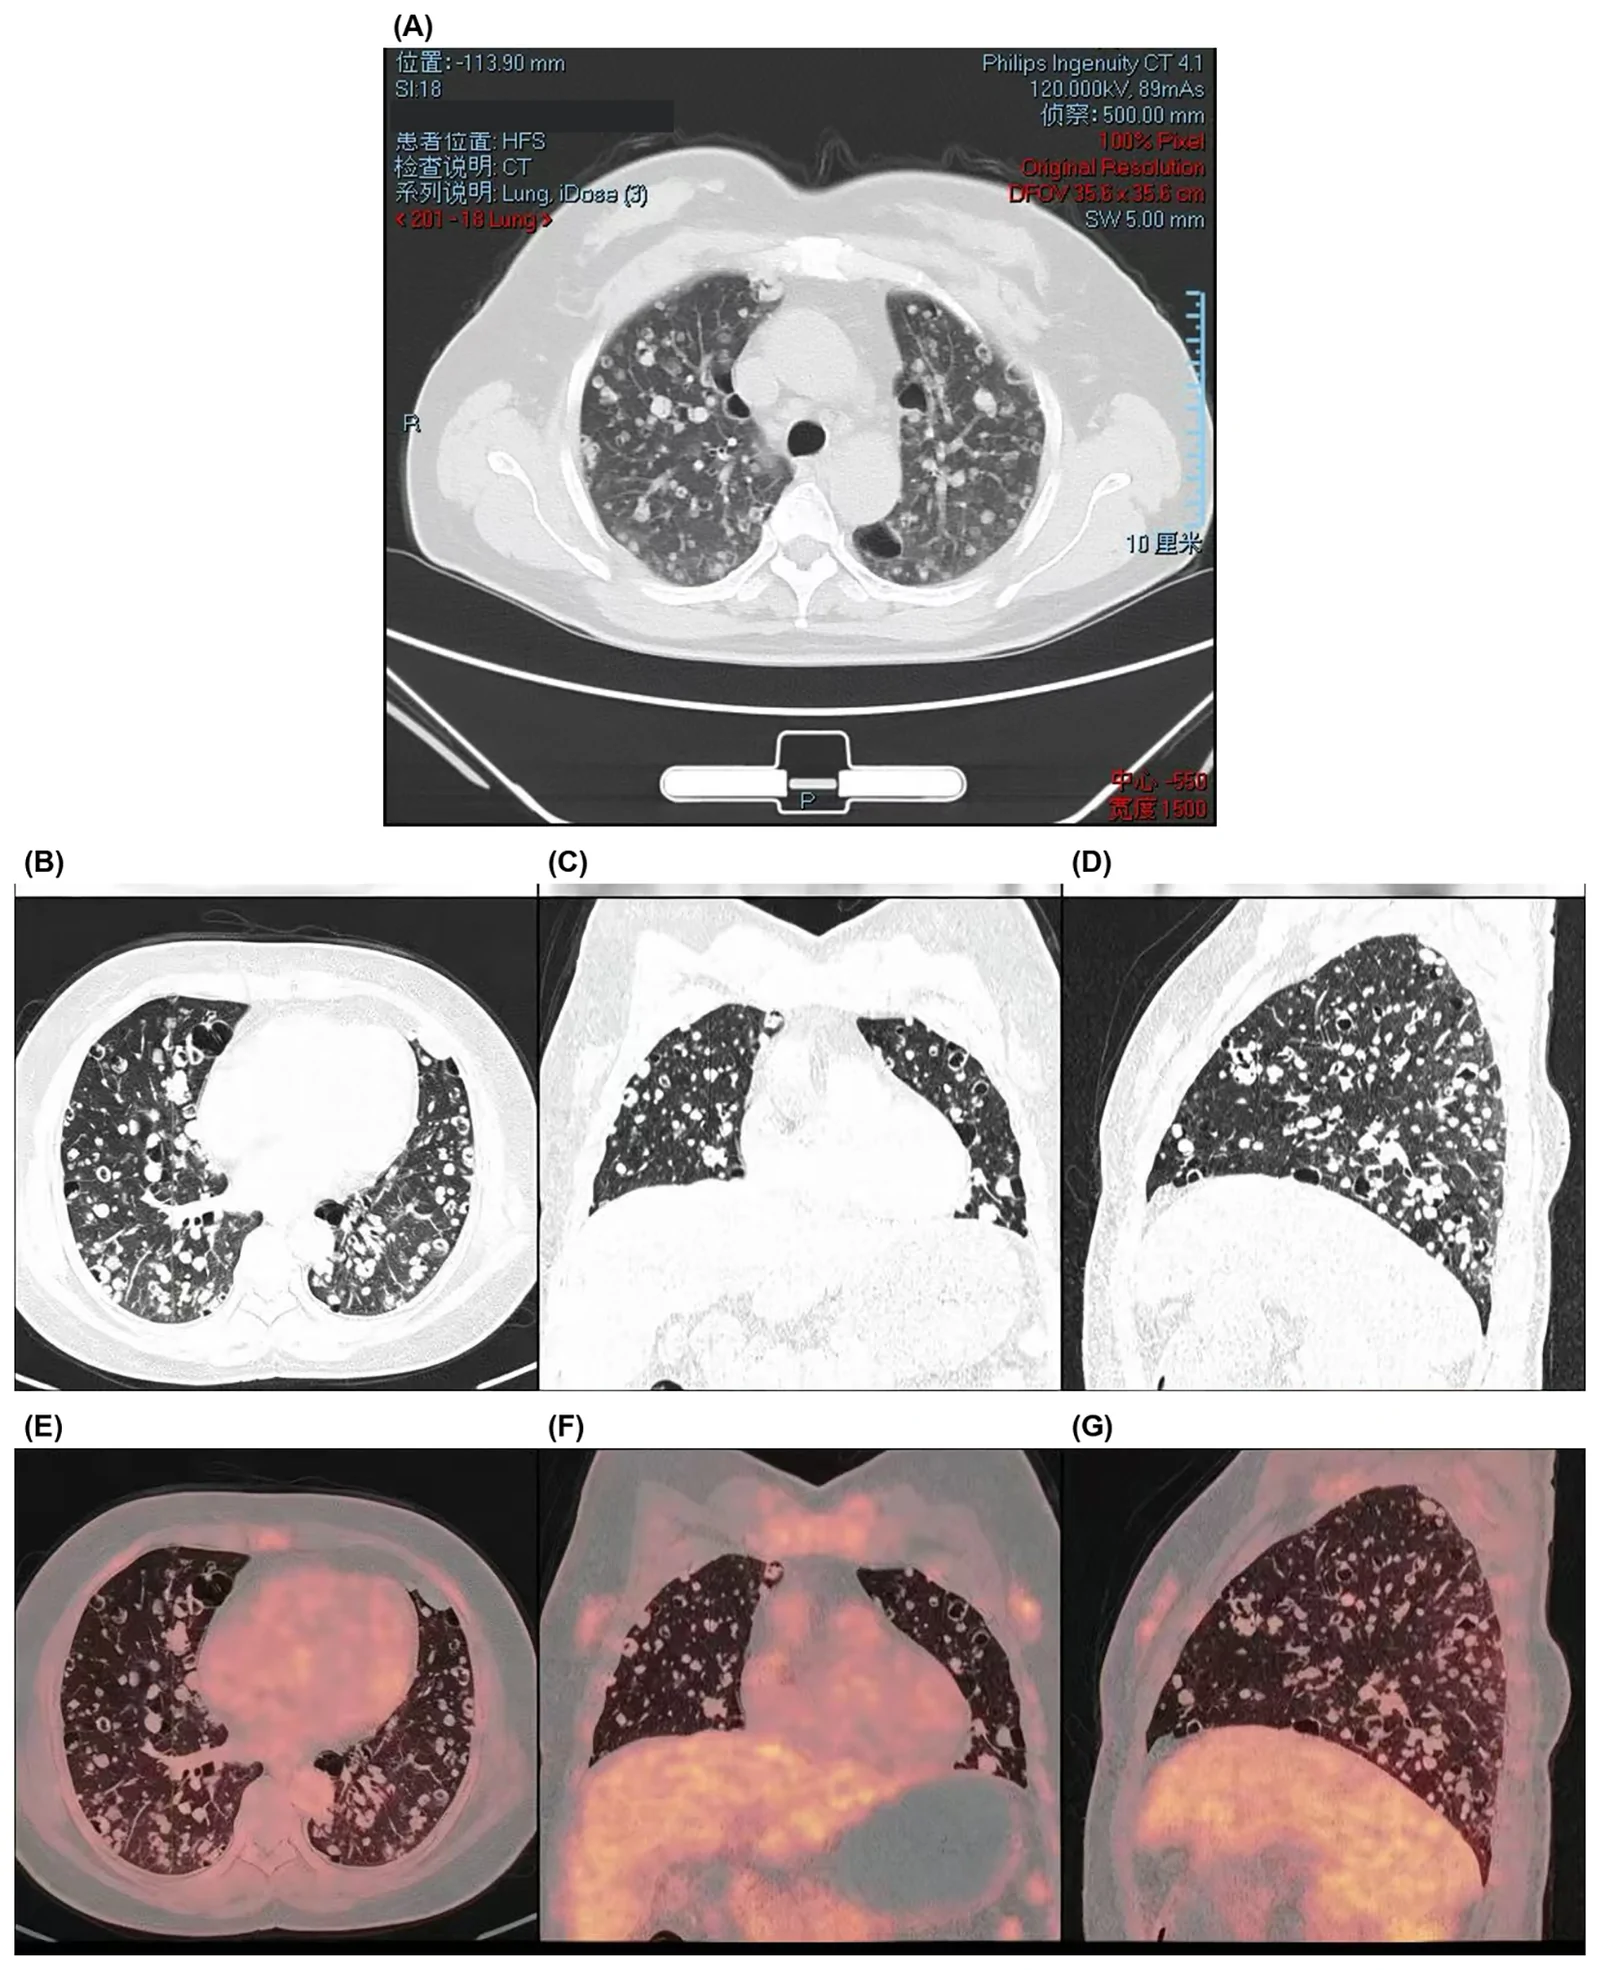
Chest CT and ^18^F-FDG PET/CT findings. (A) Representative axial noncontrast chest CT image shows diffuse bilateral pulmonary nodules admixed with thin-walled cystic or cavitary lesions. (B–D) Axial, coronal, and sagittal CT components of the PET/CT examination show the bilateral nodular-cystic distribution. (E–G) Corresponding fused ^18^F-FDG PET/CT images demonstrate mild heterogeneous FDG uptake in the dominant pulmonary lesion (SUVmax 2.44); mild nodal and diffuse bone-marrow uptake were interpreted as likely reactive changes.
